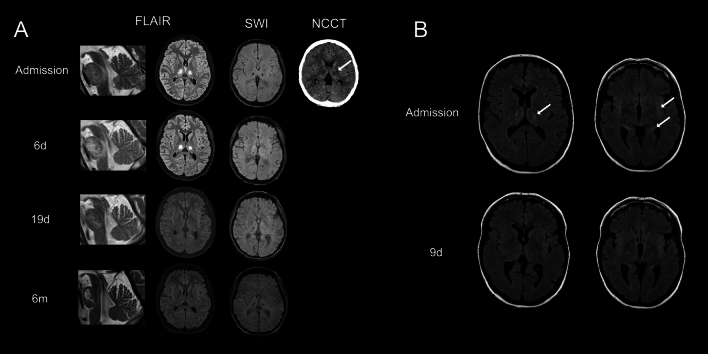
Imaging at admission and follow-ups of the index patient and her mother. A Cerebral signal alterations of the index patient are shown at onset and their development over three consecutive follow-up measurements 6 days, 19 days, and 6 months after admission. Non-contrast cranial CT (NCCT) at admission showed bilateral symmetric signal alterations, mainly in the pons and thalami. Hyperintensities in Fluid-attenuated inversion recovery (FLAIR) were visible in the medulla oblongata, pons, and bilaterally in the cerebellum, thalami, hippocampi, and external/extreme capsule with a symmetric distribution as well as high mainly precentral cortical alterations. Those FLAIR hyperintensities were in part also present with b1000 diffusion restrictions, ADC hypointensities (not shown), and signal alterations in susceptibility-weighted imaging (SWI). The follow-up MRIs showed overall regression of FLAIR lesions, diffusion restrictions, and SWI abnormalities. At the same time, a mild contrast agent enhancement in the right thalamus was still visible at 19 days post-admission (not shown). B FLAIR images at admission and 9 days follow-up of the mother show bilateral hyperintensities in the thalamus, external/extreme capsule, and parahippocampal gyrus at onset, which were almost entirely reversible over time
